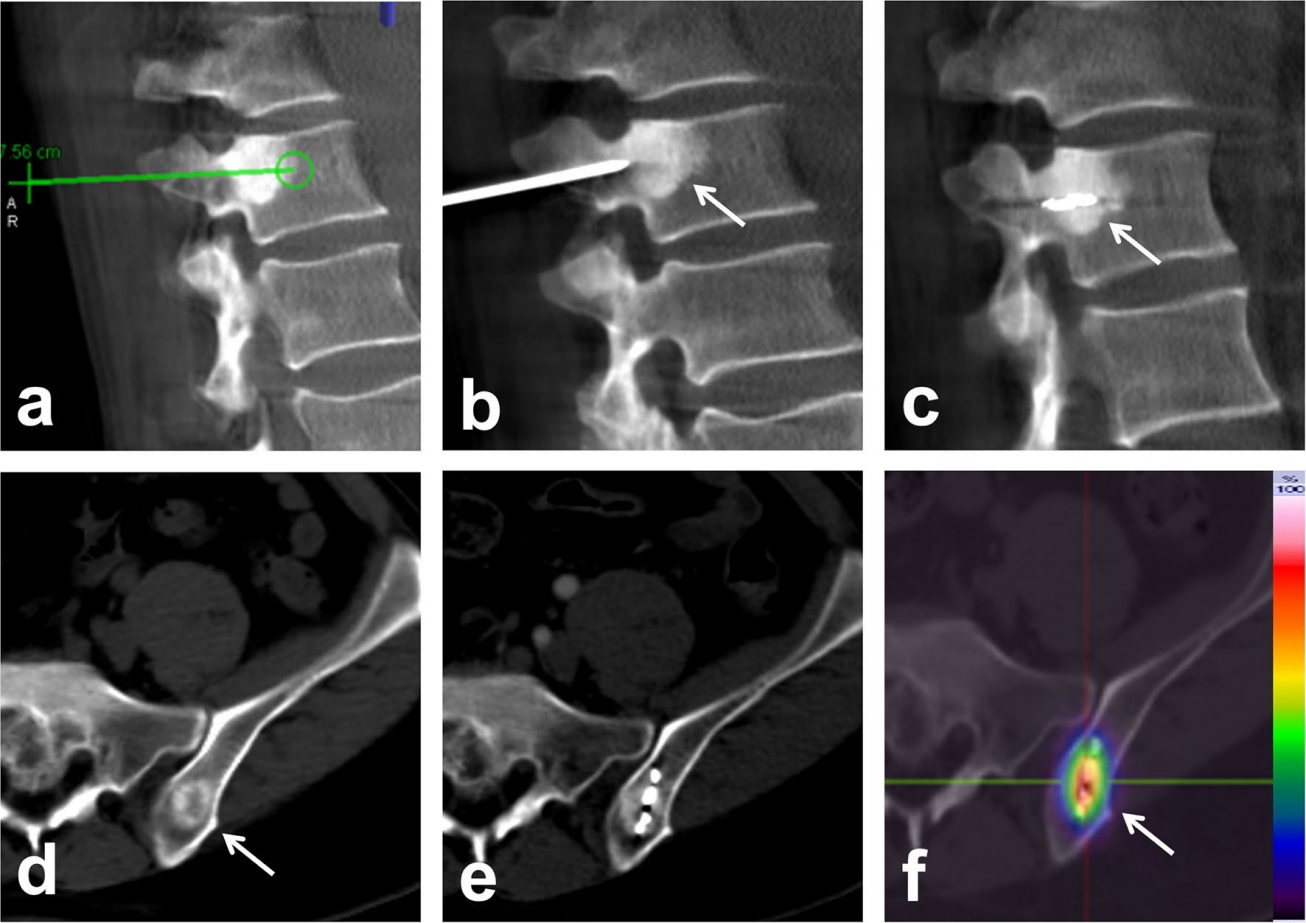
A 63-year-old female patient with lumbar spine and iliac bone metastases from breast cancer. The CT images (a–c) showed the location of the metastatic tumor foci in the lumbar spine, the virtual puncture path, and guidance of  the puncture and ISI. CT images (d–e) showed the metastatic tumor in the iliac bone and the implanted seeds. Image (f) showed the radioactive distribution of the seeds chain after ISI
